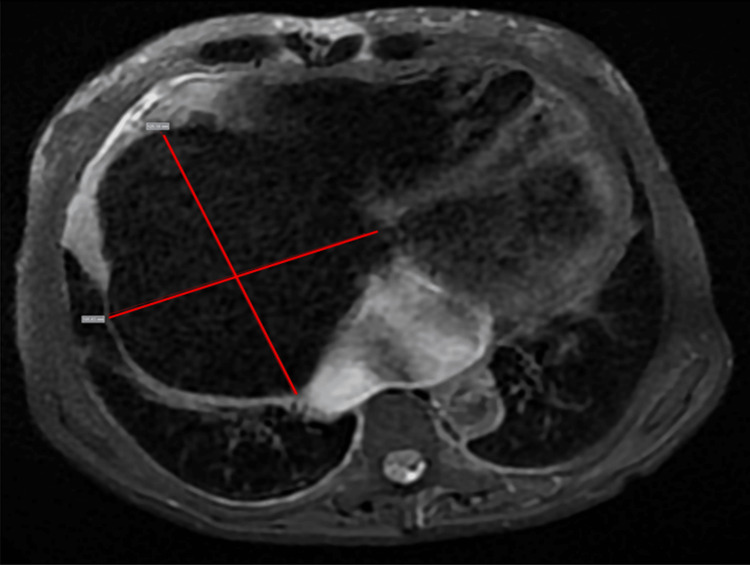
MRI scan. Axial T2-weighted scan with fat saturation shows a severely enlarged right atrium, measuring 12.5 x 10.5 cm (anteroposterior and transverse diameters).
